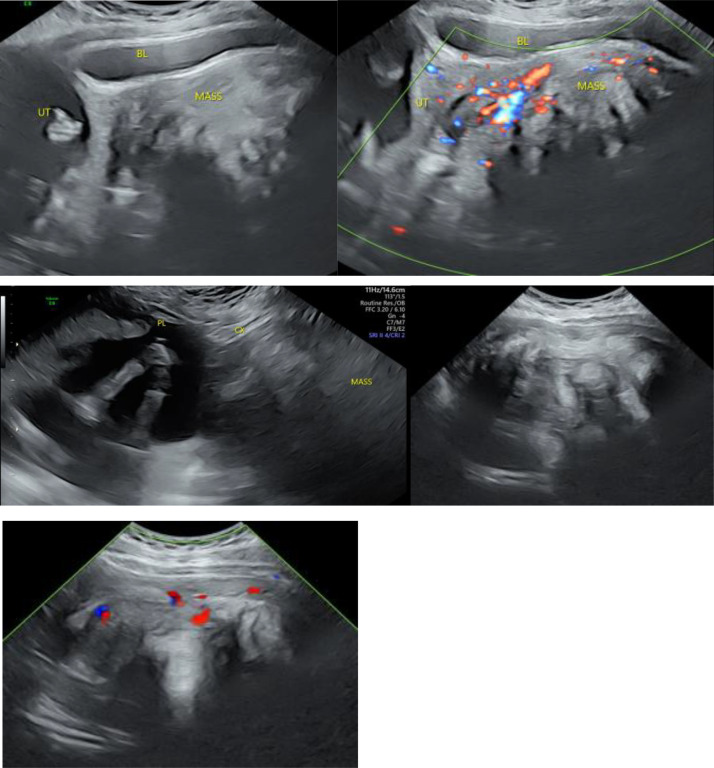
Transperineal grayscale and color Doppler ultrasound images of the pelvis showed a large, heterogeneous mass with posterior acoustic shadowing and significant vascularity, which was later diagnosed as AAM. It displaced the gravid uterus, cervix, and bladder superiorly. The mass could not be fully measured due to its massive size. Key: MASS = AAM, BL = bladder, UT = uterus, CX = cervix, PL = placenta.
